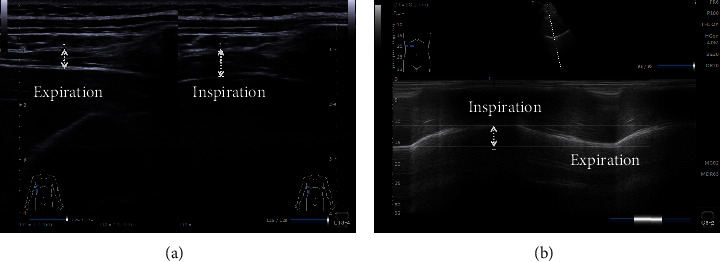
Ultrasound measurements of diaphragm. (a) Diaphragm thickness in B-mode. (b) Diaphragm excursion in M-mode.
